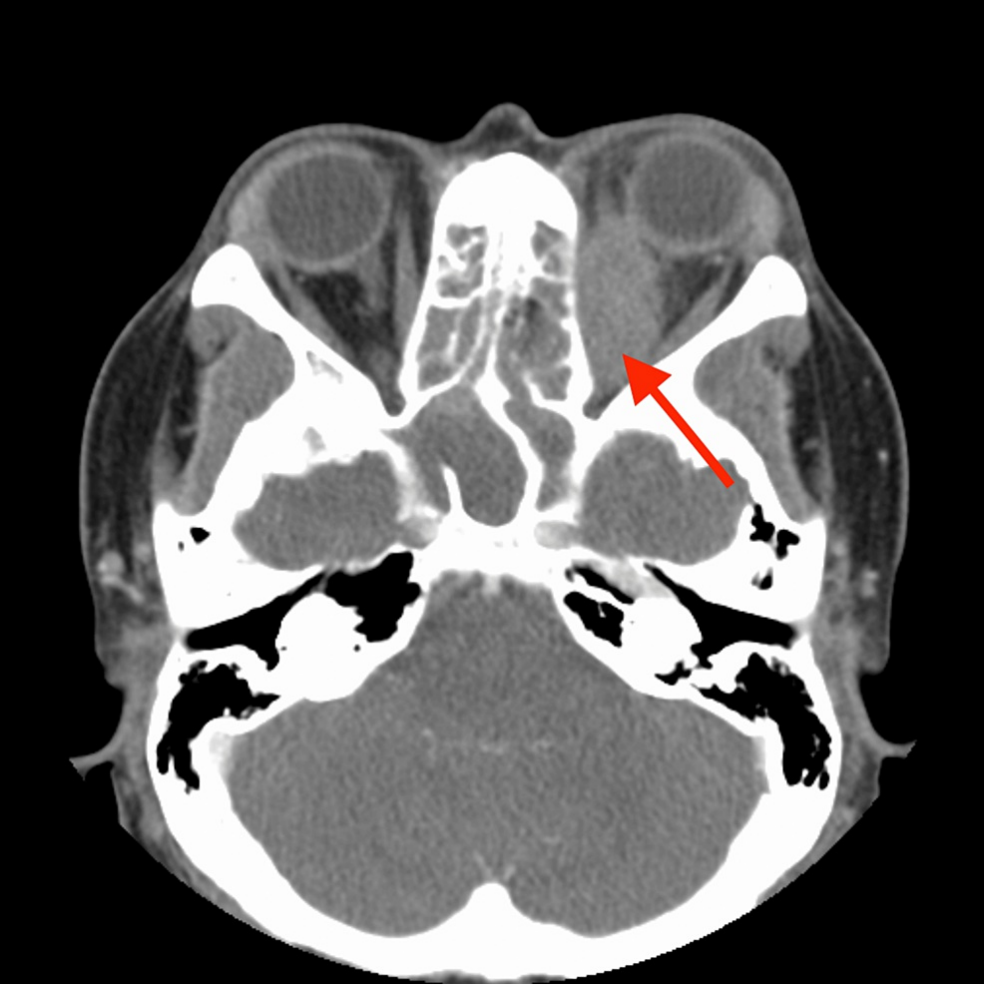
CT facial bones with contrast axial CT: computed tomography
